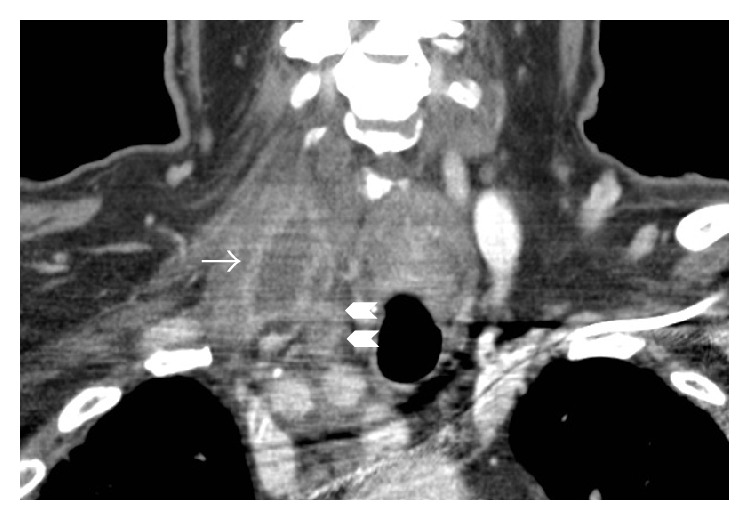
Coronal CT demonstrating a rim-enhancing fluid collection in the right neck (arrow) and inflammatory changes tracking into the mediastinum (chevrons).
